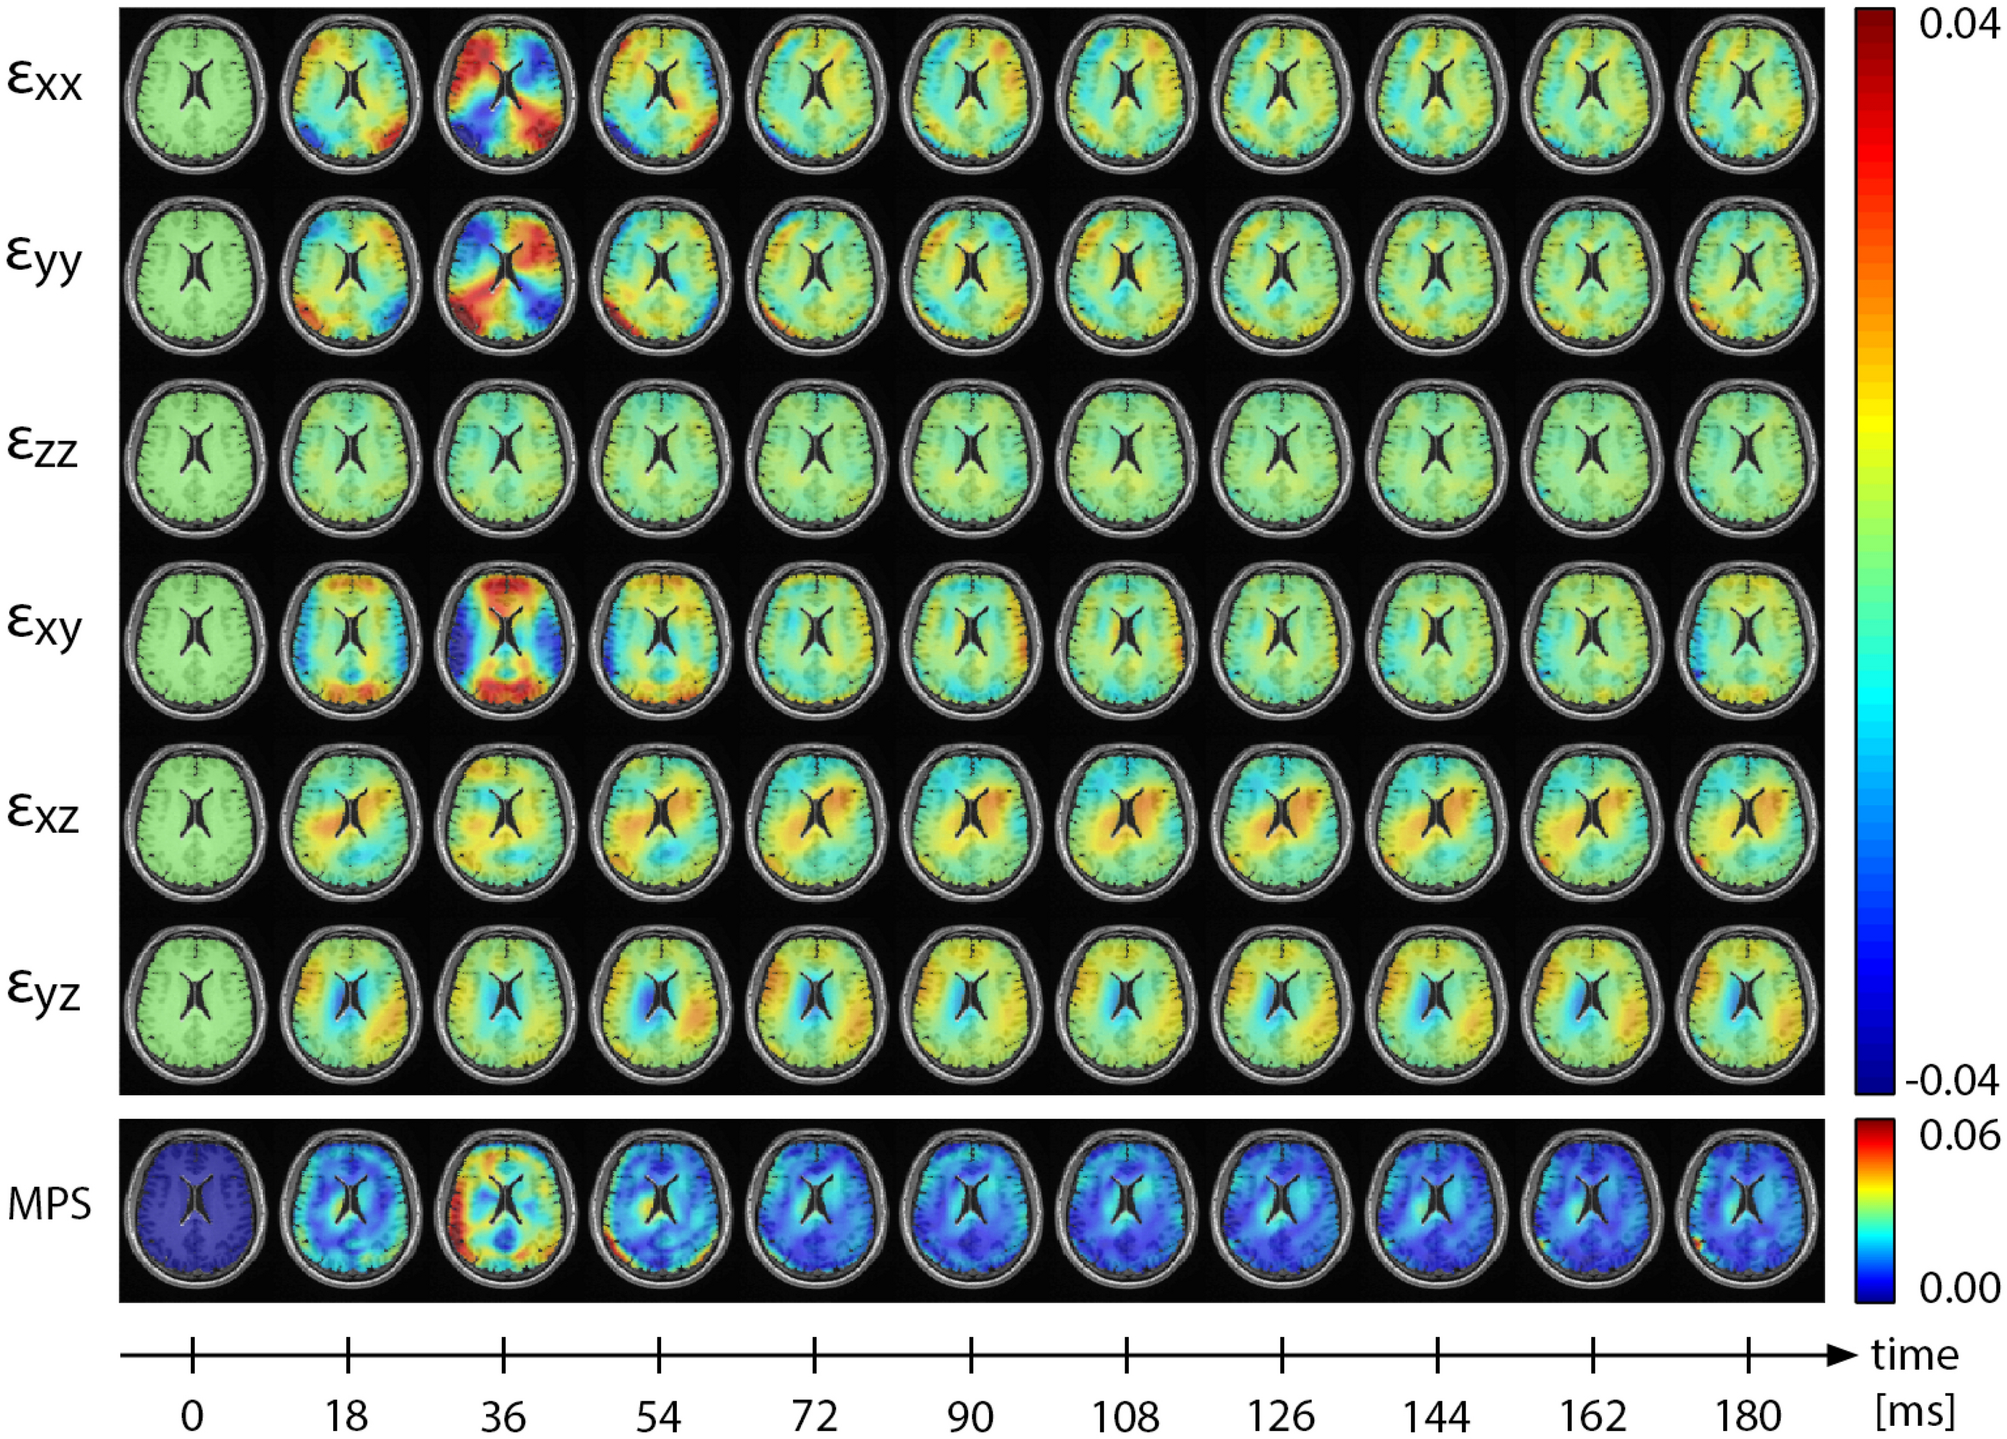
Deformation (strain) in the brain of a human volunteer, measured with tagged MRI during its response to impulsive angular acceleration of the head. All components of the strain tensor (*ε*_*ij*_) are captured throughout the entire brain and across time. These strain fields can be used to calculate summary strain metrics, such as maximum principal strain (MPS).
